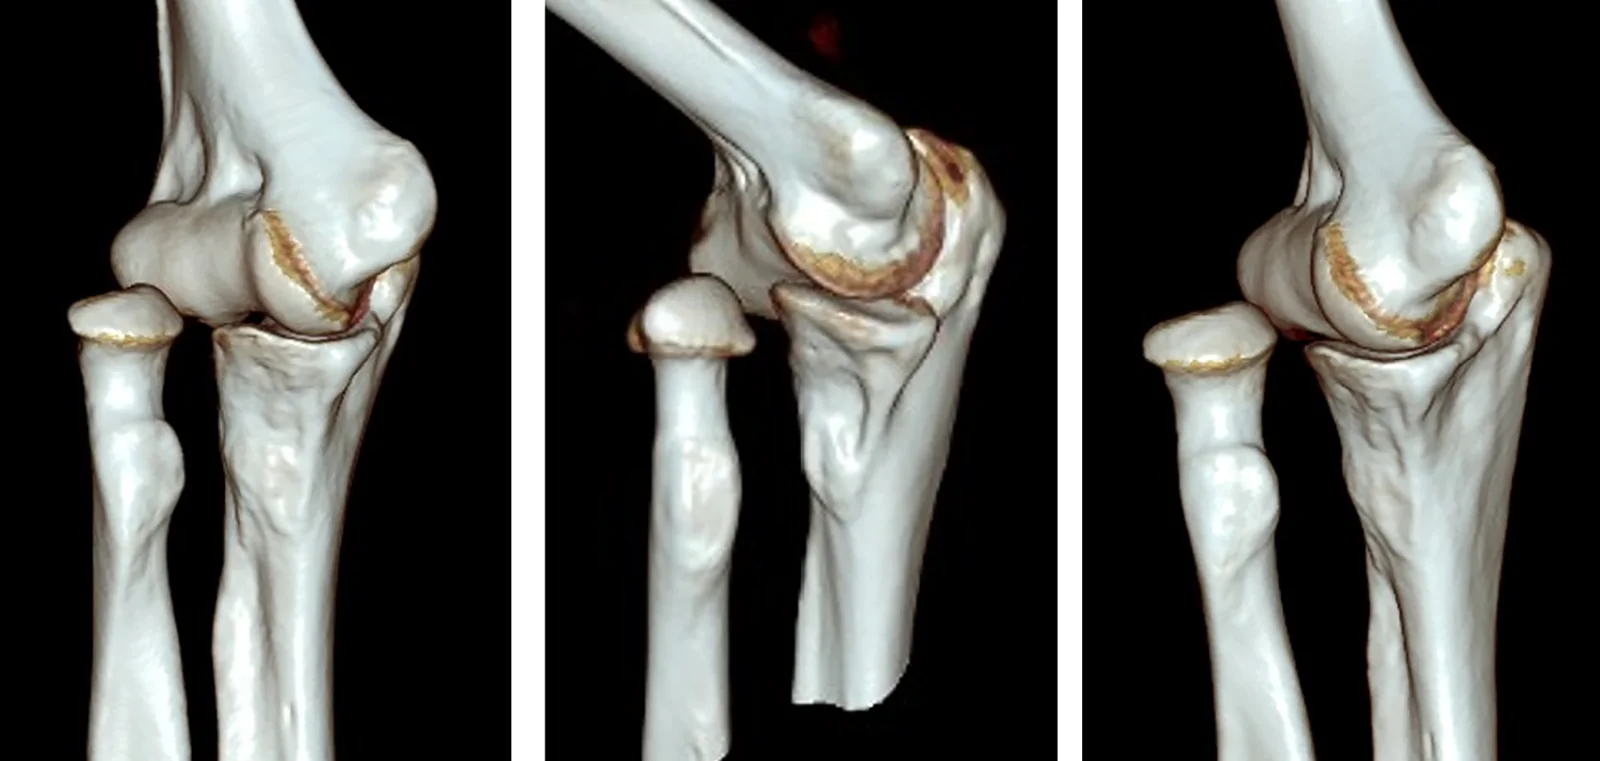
Preoperative computed tomography (CT) images showing chronic radial head dislocation with severe radial head deformity and incongruent radiocapitellar articulation.
